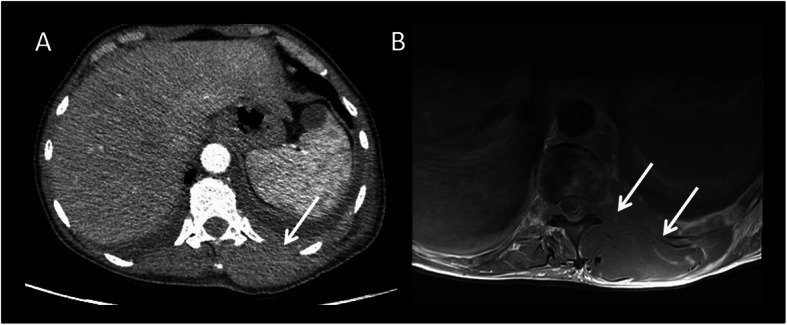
Patient affected by non-Hodgkin lymphoma. a CT imaging shows a lesion that infiltrates the left paravertebral muscle with extension in the paravertebral space. b MRI confirms an infiltrative mass with substitution of the left paravertebral muscle, the left adipose paravertebral space, and the posterior costal arch. This type of growth and behavior is strongly suggestive for a localization of lymphomatous disease
